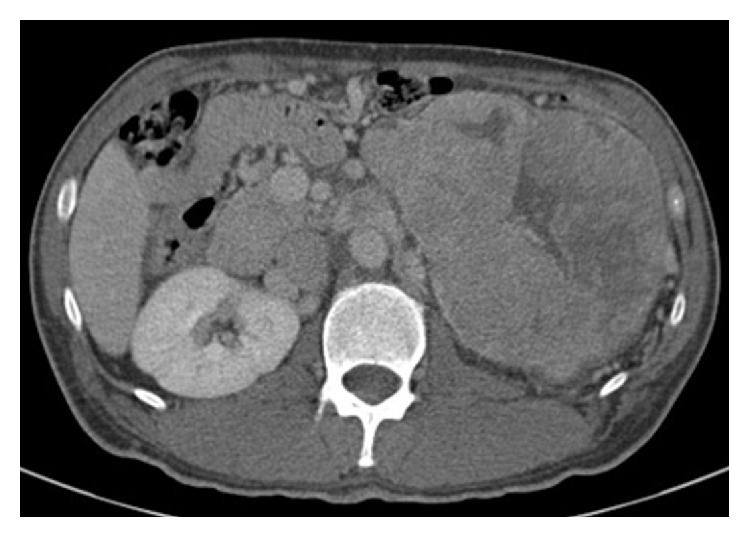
Abdominal computed tomography demonstrating a heterogeneously enhanced mass, 14 × 12 cm in diameter, with internal necrotic changes in the left adrenal region.
